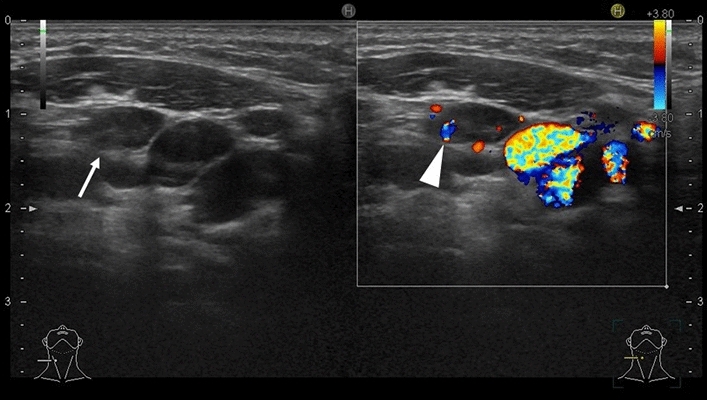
Ten-year-old girl had fever and palpable node at neck. Ultrasonography revealed low echoic nodule with linear high echogenicity (hilum) (arrow). Doppler US depicted flow at hilum of lymph node (arrow head). These are findings of normal lymph nodes.
